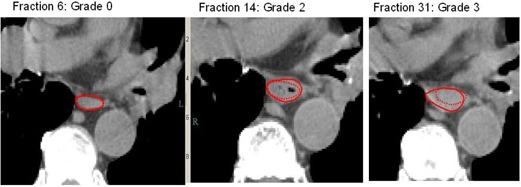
Weekly CT images for Patient A. The dotted contour shows the shape of the esophagus in the treatment plan. The solid contour shows the current shape of the esophagus, from deformable registration. Expansion of the esophagus can be seen from fraction 14, corresponding with the esophagitis grade in the patient record.
